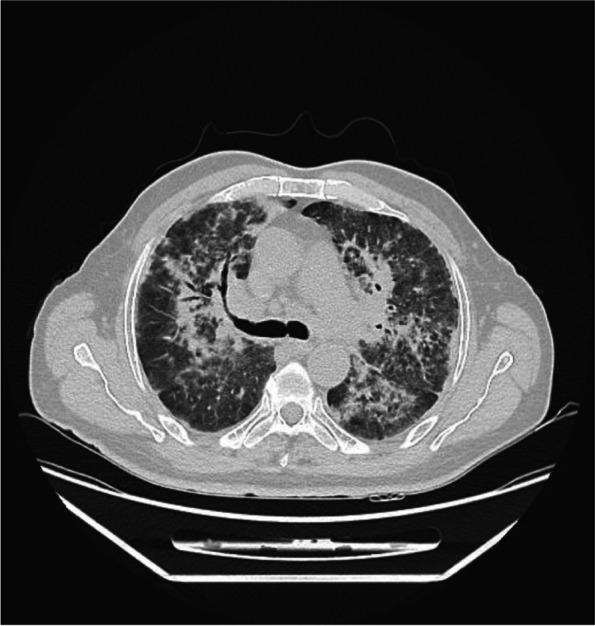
High-resolution computed tomography of the chest showing patchy bilateral areas of consolidations and ground-glass opacities with early changes of fibrosis
